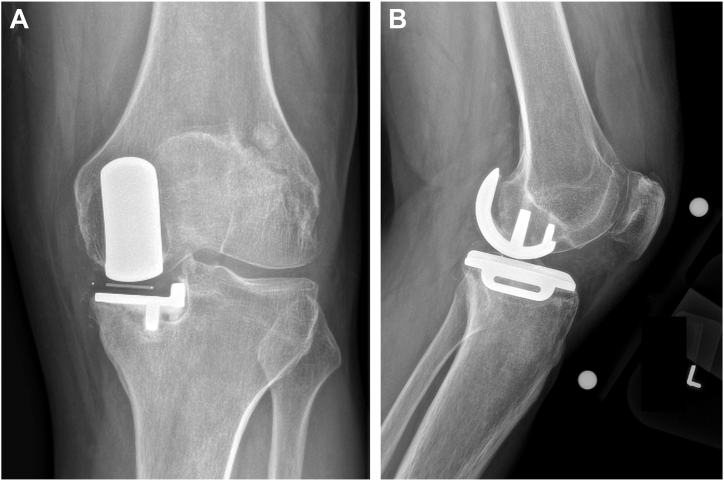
Preoperative anteroposterior (a) and lateral (b) radiograph of a failed unicompartmental knee arthroplasty.
